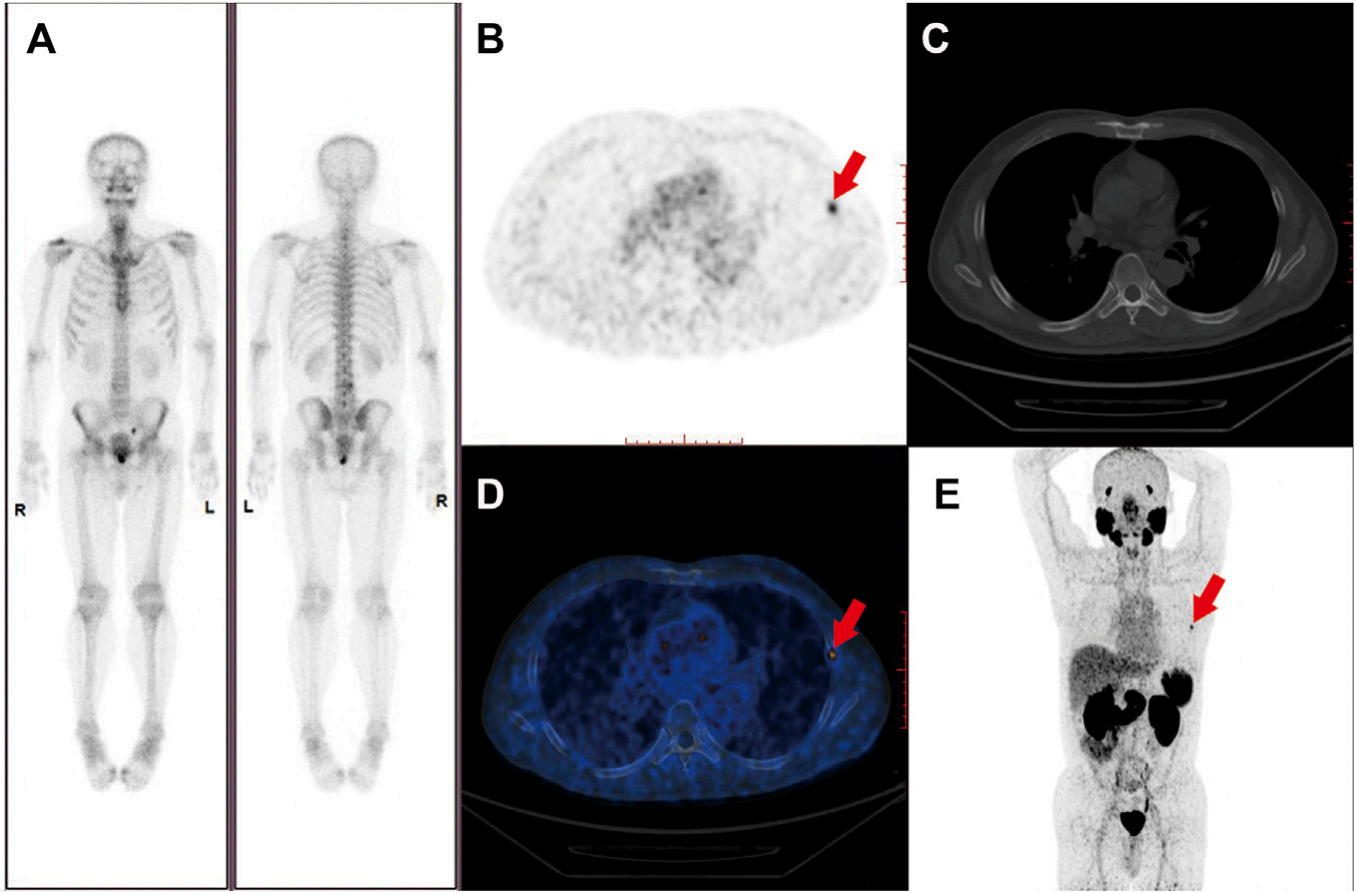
Imaging of a 56-year-old patient with Gleason score 7 prostate cancer and rising PSA following laparoscopic prostatectomy. PSA was 1.51 ng/ml at the time of the PET scan. PSMA-PET maximum-intensity projection, axial slides, and axial fused PET/CT revealed metastasis bone lesion on the left fifth rib (where the red arrows pointed to) with a SUVmax of 5.1 (B,D,E). The lesion was not detected on the (A) Bone scan and (C) CT scan performed simultaneously.
